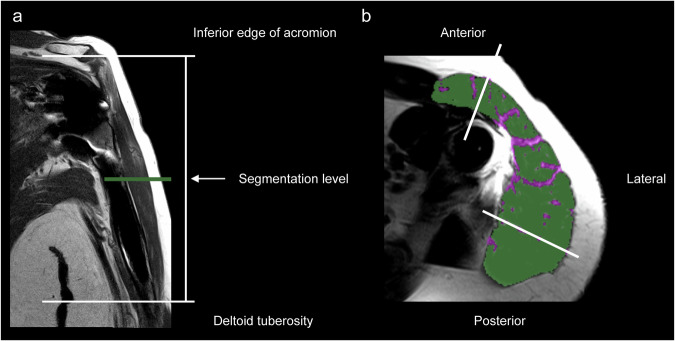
Deltoid muscle segmentation method. a Coronal T1w MRI sequence showing the selected measurement level (green line) for axial analysis at the mid-level between the inferior edge of the acromion and deltoid tuberosity. b Corresponding axial T2w sequence with WARP metal artifact reduction demonstrating segmentation of lean deltoid muscle (green overlay) and intramuscular fat (purple overlay), with anatomical subdivision into anterior, lateral, and posterior parts. Note that despite metal artifact reduction, susceptibility artifacts are still visible around the implant components, but do not significantly affect the deltoid muscle analysis at this level
